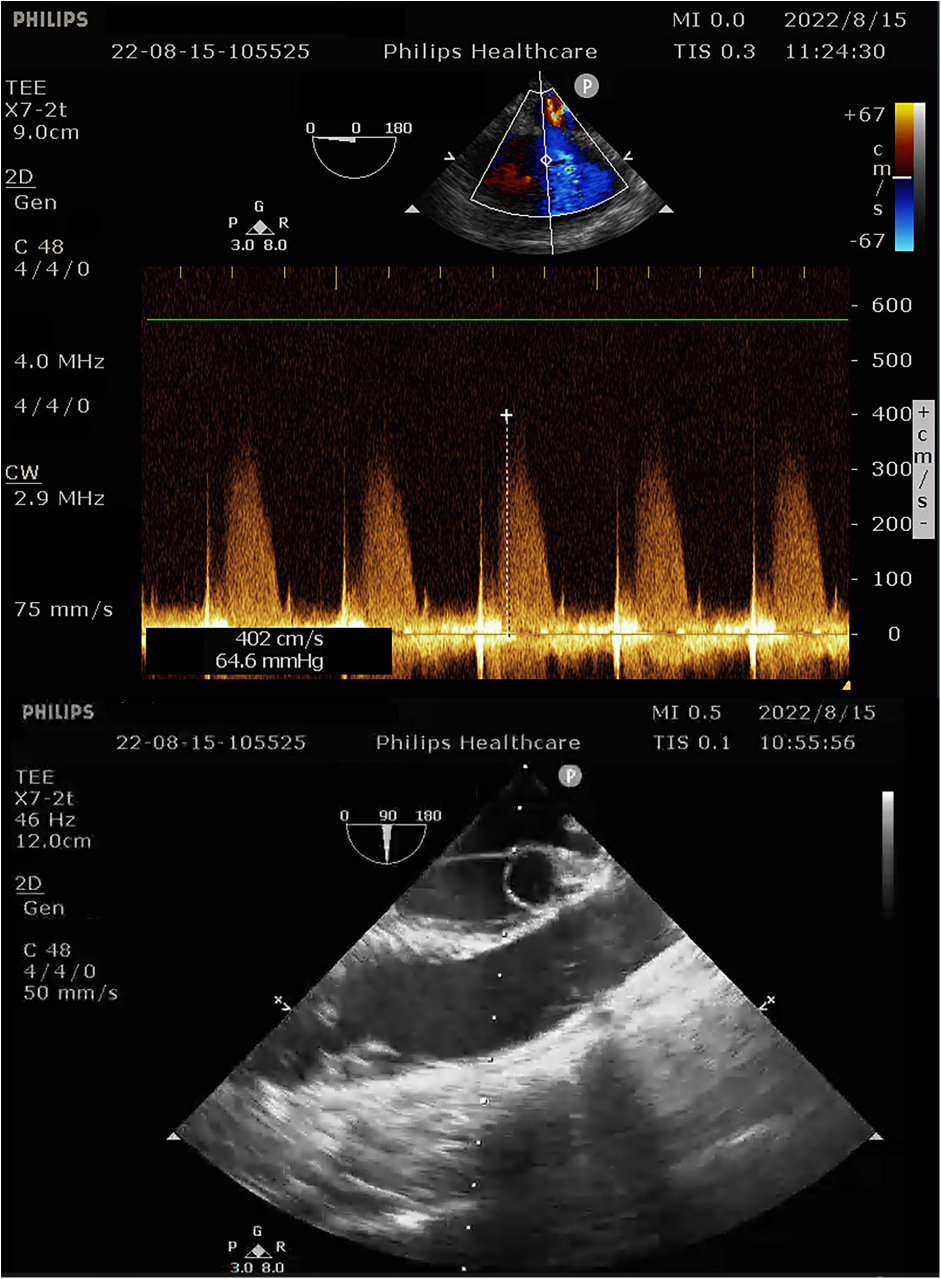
Intra-operative transesophageal ultrasound indicating pulmonary artery pressure of 69 mmHg.
